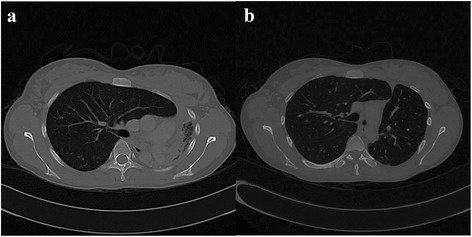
Axial view of a CT Thorax at the main carina level pre- and post-intervention. a CT Thorax showed left main bronchus stenosis with collapsed left lung. b CT Thorax at 36 weeks following intervention with residual left main bronchus stenosis and re-expansion of the left lung
